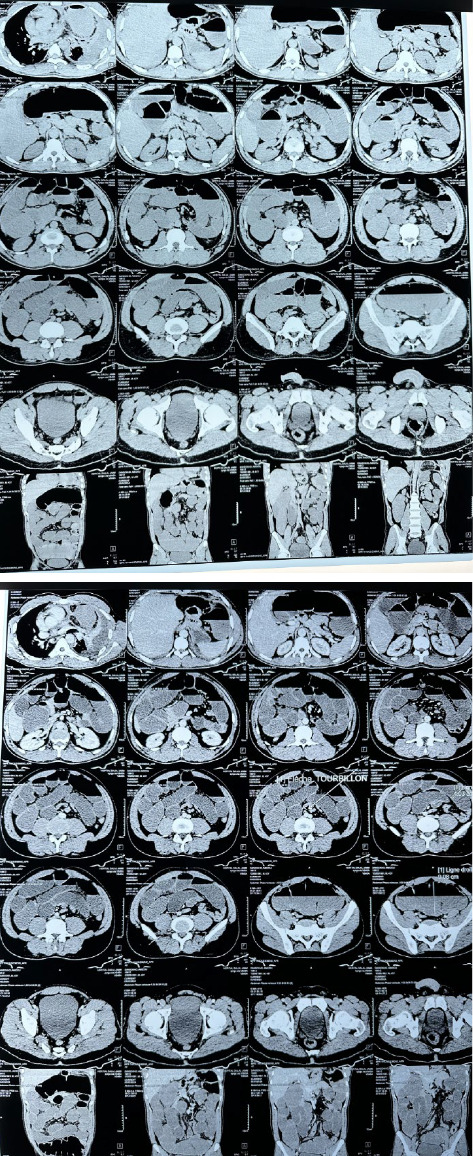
Contrast-enhanced CT scan of the abdomen and pelvis, in both axial and frontal views, showing distended bowel loops, a pattern of mesentero-axial volvulus of the colon, and a moderate peritoneal effusion.
